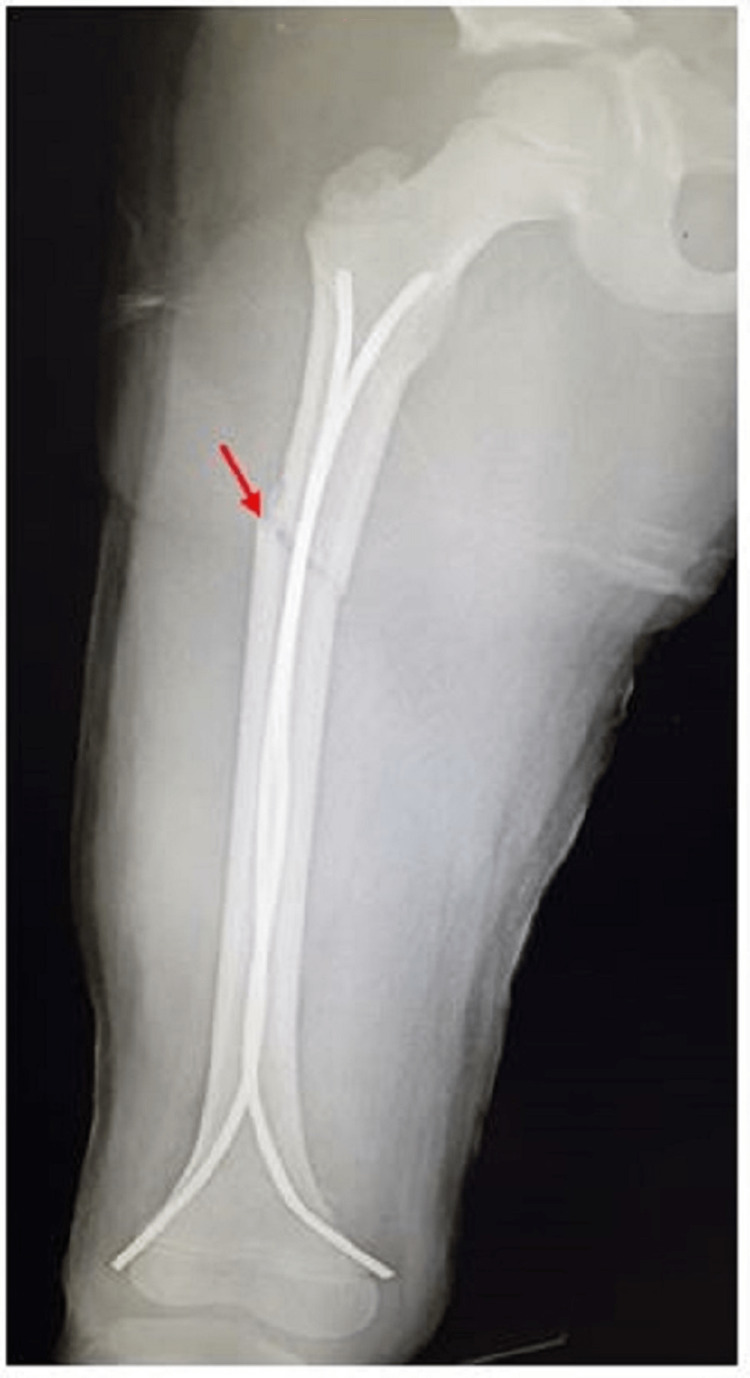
Antero-posterior radiograph of a nine-year-old male with right subtrochanteric fracture (red arrow) who underwent TENS fixation TENS: titanium elastic nailing system
